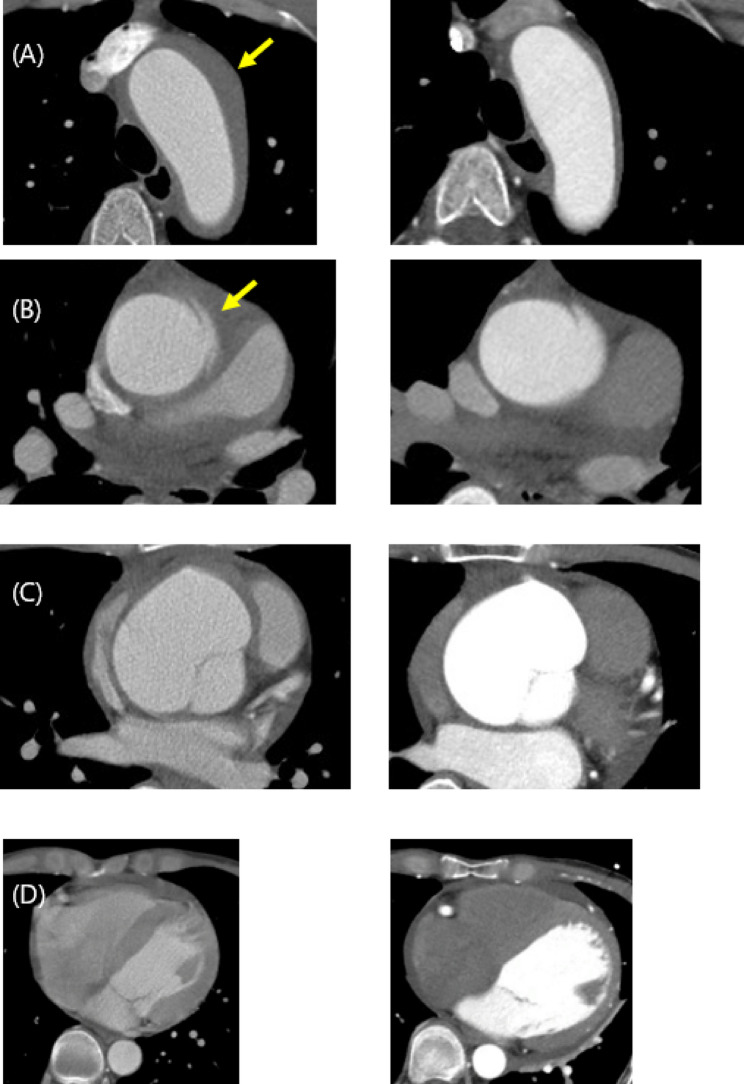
Comparison between initial and follow-up contrast-enhanced CT images. The left column shows the initial CT at presentation, and the right column shows the follow-up CT obtained on day 3 at corresponding anatomical levels. (A) Blood tracking along the aortic arch on the initial CT, (arrow), which resolved on follow-up imaging., (B) Serial axial images demonstrating progression of the aortic contour abnormality in the proximal ascending aorta; a subtle contour bulge on the initial CT, (arrow) becomes more conspicuous on follow-up imaging., (C) Dilatation of the aortic root measuring 60 mm, without significant interval change., (D) Mild pericardial effusion, which remained stable on follow-up imaging
